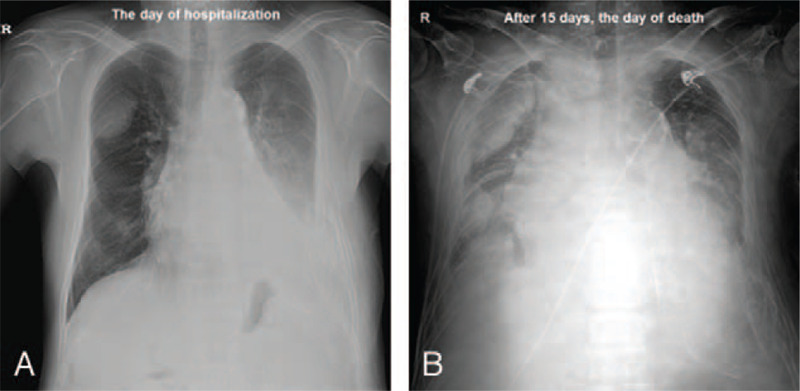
Serial simple radiography showed rapid progression of the tumor in the right hemithorax and pulmonary edema, when comparing images performed on the day of hospitalization (A) and the day of death (B).
